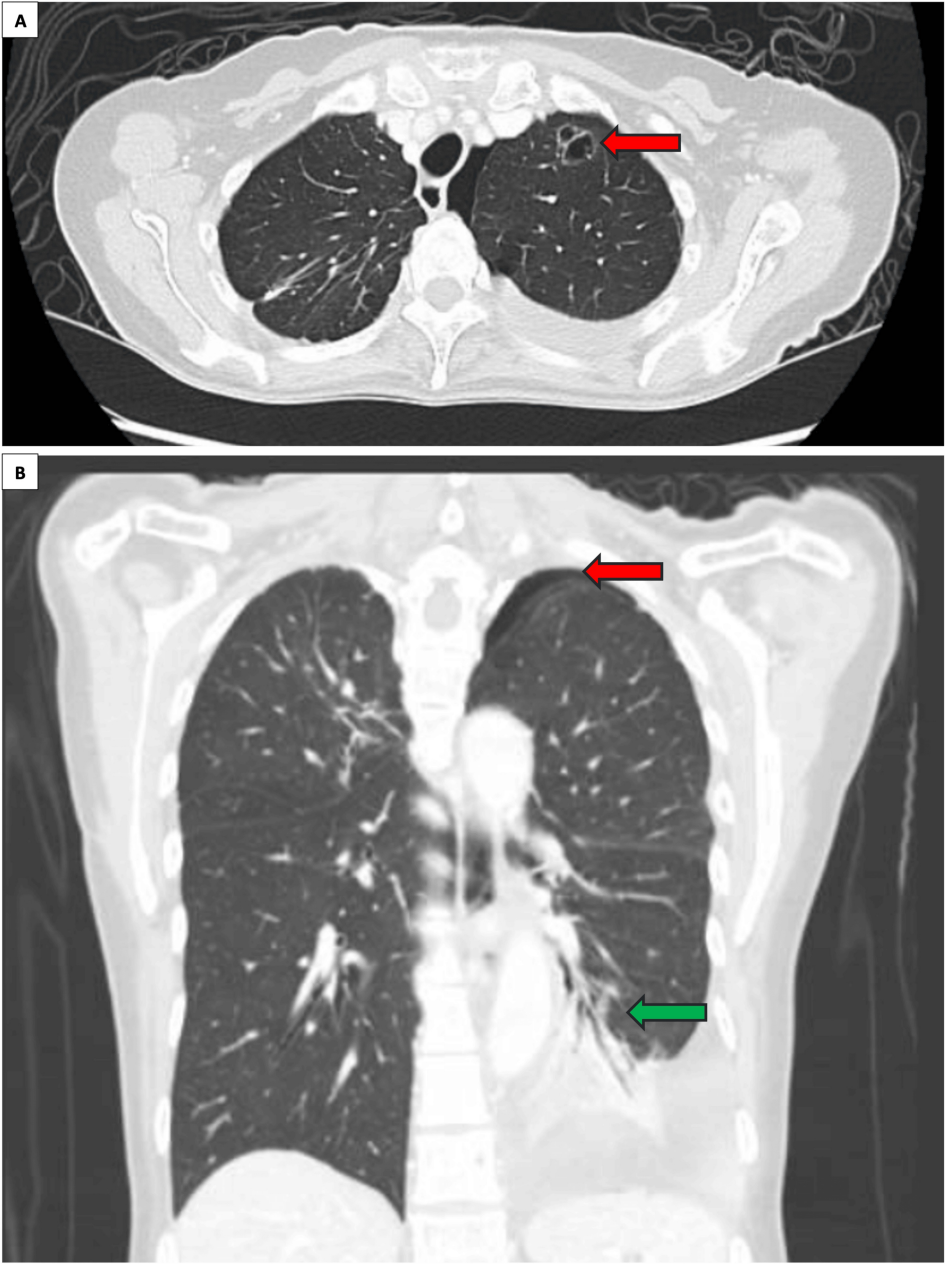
(A) Axial cut of the chest CT six months later, which reveals an interval increase in size of the left apical cystic lesion, from 1 cm to 1.9 cm (red arrow). (B) Coronal cut of the chest CT showing moderate left hydropneumothorax (red arrow) with partial collapse of the left lower lobe and adjacent compressive atelectasis (green arrow). CT: computed tomography
